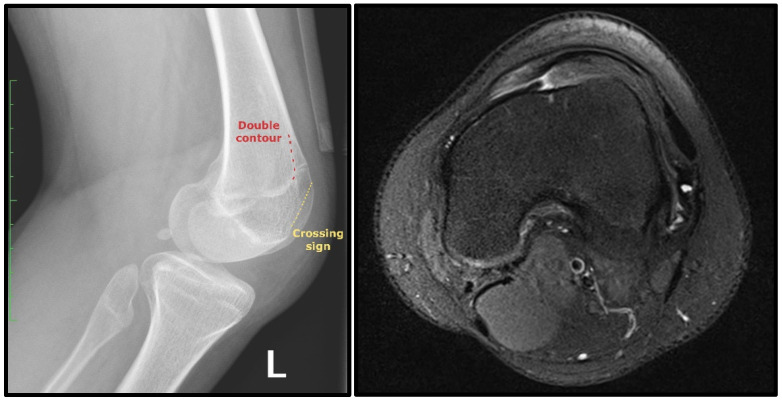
Lateral knee radiograph and axial MRI showing signs of trochlear dysplasia consistent with Dejour type B trochlear dysplasia. Radiograph with crossing sign and double contour. MRI shows a hypoplastic medial facet, whereas the lateral aspect of the trochlea appears bulged.
